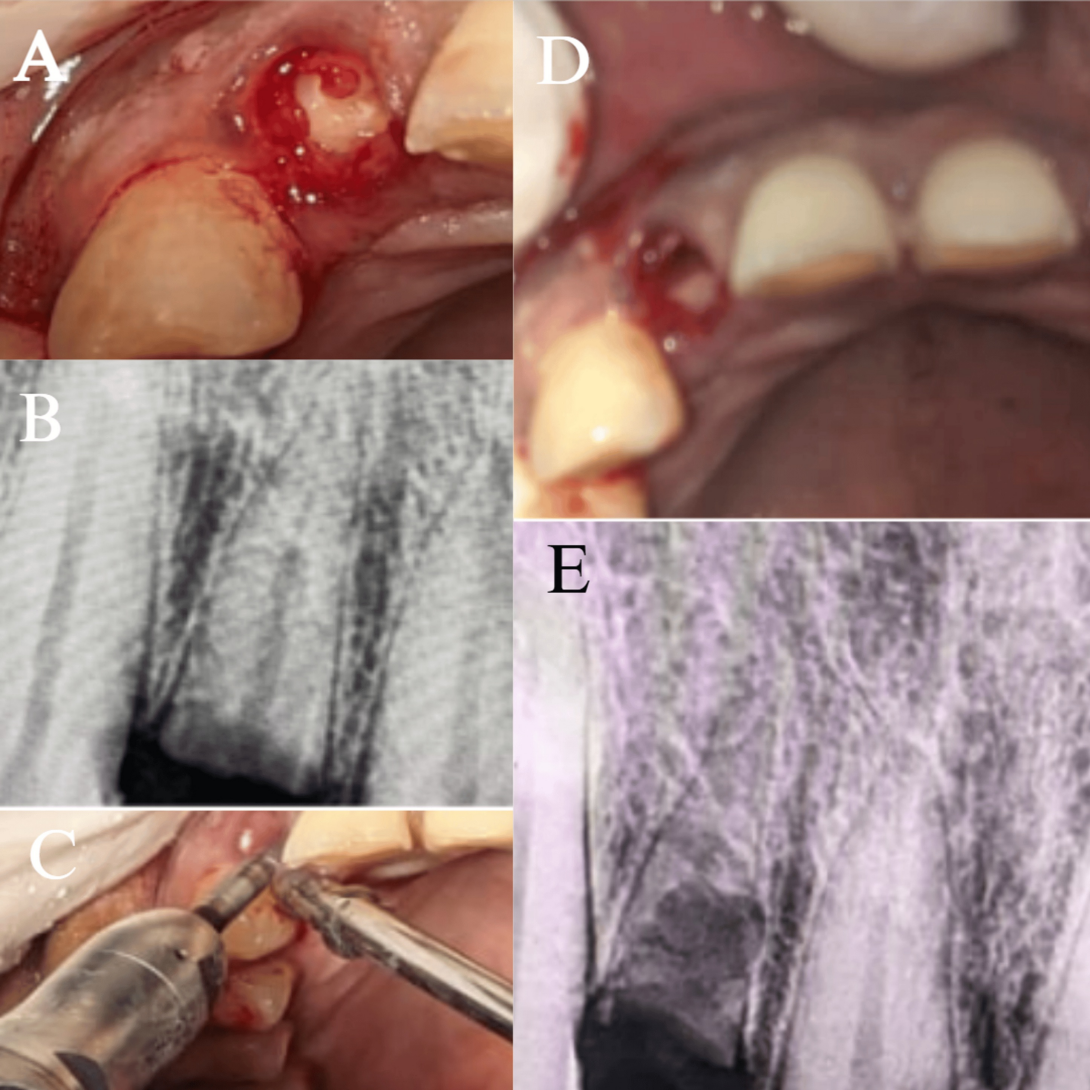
(A) Exposing the root segment of #12; (B) radiograph of the root segment; (C) sectioning of the root segment; (D) intraoral image of the labial root segment; (E) radiograph of the sectioned labial root segment (A) shows the clinical image of the root of #12, whereas (B) shows the radiographic image of the root portion of #12. (C) shows the sectioning of the root portion; (D) shows the intraoral image of the labial segment of the root after removal of the palatal portion after sectioning; (E) shows the radiographic image of the remaining labial portion of the root of #12
